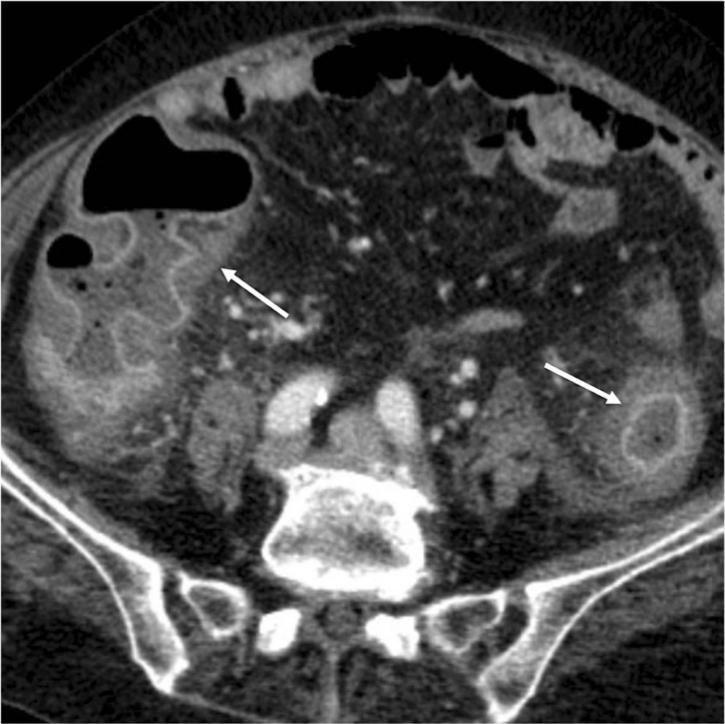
Stratified attenuation pattern in pseudomembranous colitis. Axial contrast-enhanced CT scan shows significant wall thickening of the ascending and descending colon (*arrows*) due to submucosal oedema, resulting in the stratified appearance. The marked thickening of the bowel wall and the mild pericolonic fat stranding suggest pseudomembranous colitis
